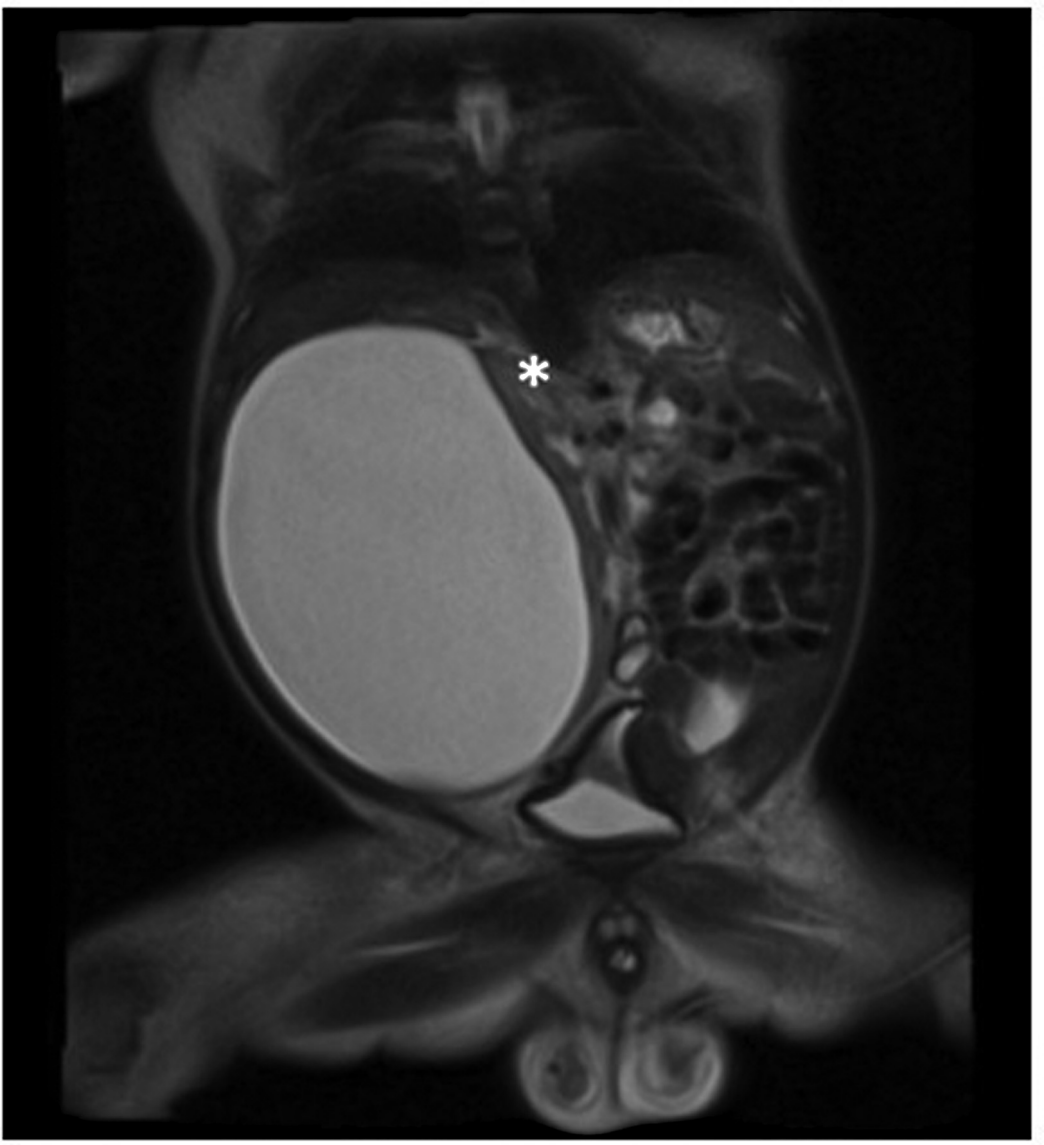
Magnetic resonance imaging at second day of life, asterisk indicating upper pole of right kidney.
